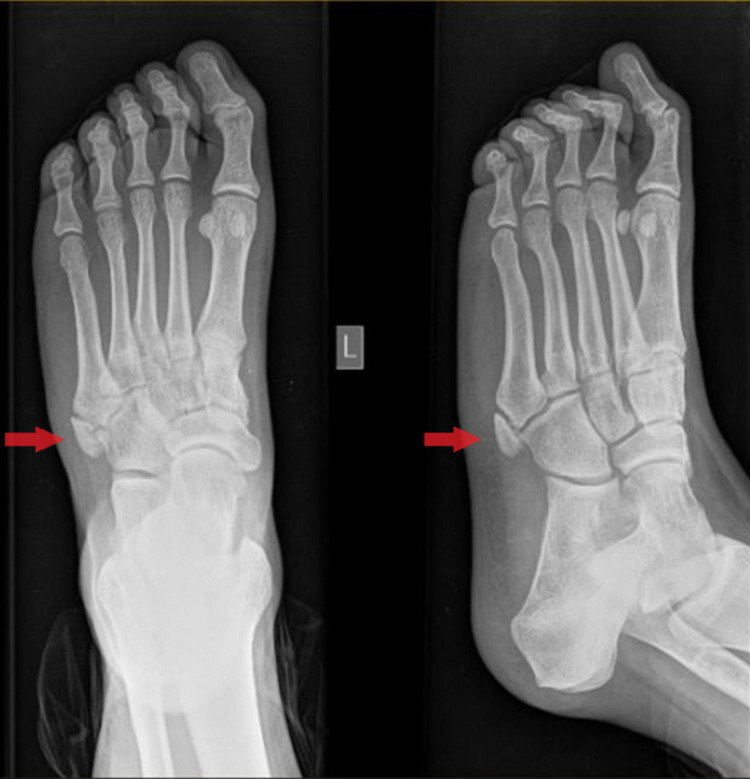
Plain radiograph (AP and lateral oblique) of the left foot reveals the presence of the os vesalianum pedis articulating with both the base of the fifth metatarsal and the cuboid bone (red arrow). AP: anteroposterior
